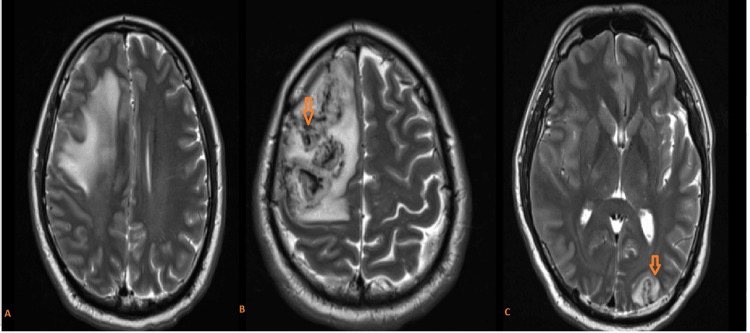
MRI brain axial T2-weighted (T2W) imaging (A, B, C) A, B: T2W imaging suggestive of large heterogeneously hyperintense signal intensity at the right frontal lobe (arrow) C: Mixed-intensity lesion is also noted at the left occipital region (arrow)
